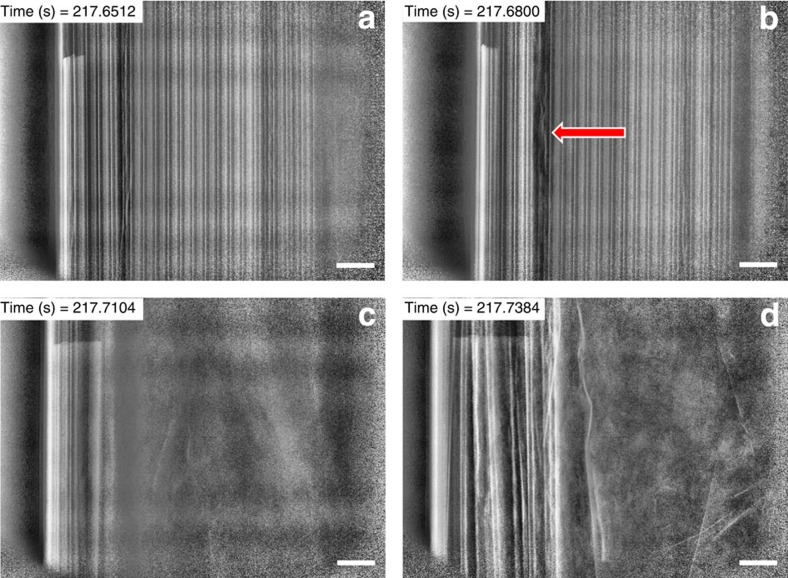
Radiographs from Supplementary Movie 6 showing the propagation of thermal runaway in Cell 2. (a) Radiograph of the *YZ* plane before thermal runaway; (b) radiograph during thermal runaway where the red arrow indicates the region in which structural breakdown is first observed; (c) radiograph during ejection of contents; (d) radiograph immediately after ejection of contents. The time-stamped radiographs show that the entire process of initiation and ejection lasted <0.1 s. Heating is applied from the right of the images, but continuous rotation at 180° every 0.2 s maintains an even circumferential temperature distribution. Scale bar, 1 mm.
